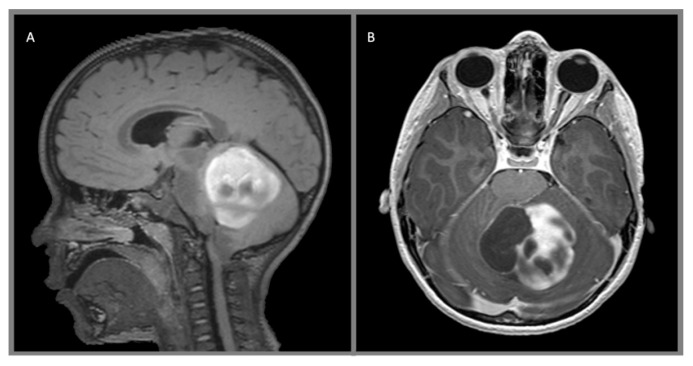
Juvenile pilocytic astrocytoma, WHO 1: (A) sagittal T2 FLAIR with contrast and (B) axial T1 with contrast demonstrate a heterogeneous, multicystic, avidly enhancing mass arising from the left cerebellar hemisphere.
